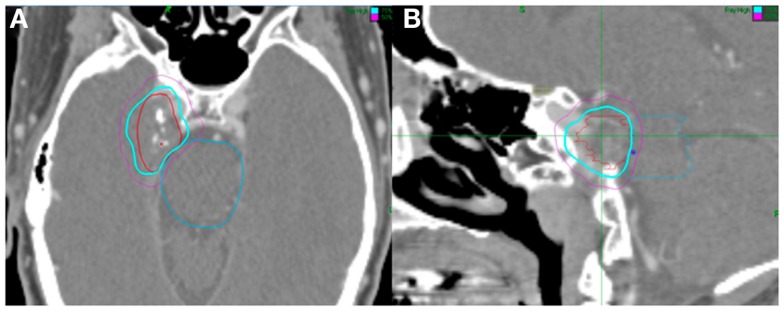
Fifty-three-year-old man with a right Meckel cave meningioma. He presented with right facial pain. The decision was to proceed with radiosurgery. Treatment planning axial (A) and sagittal (B) computed tomography images demonstrating the GTV (red), brainstem (blue), and chiasm (yellow). Isodose lines shown as follows: blue 79% (prescription) and purple 50%. Note proximity of the meningioma to the brainstem. The tumor was treated with 2500 cGy in five fractions and his pain resolved.
